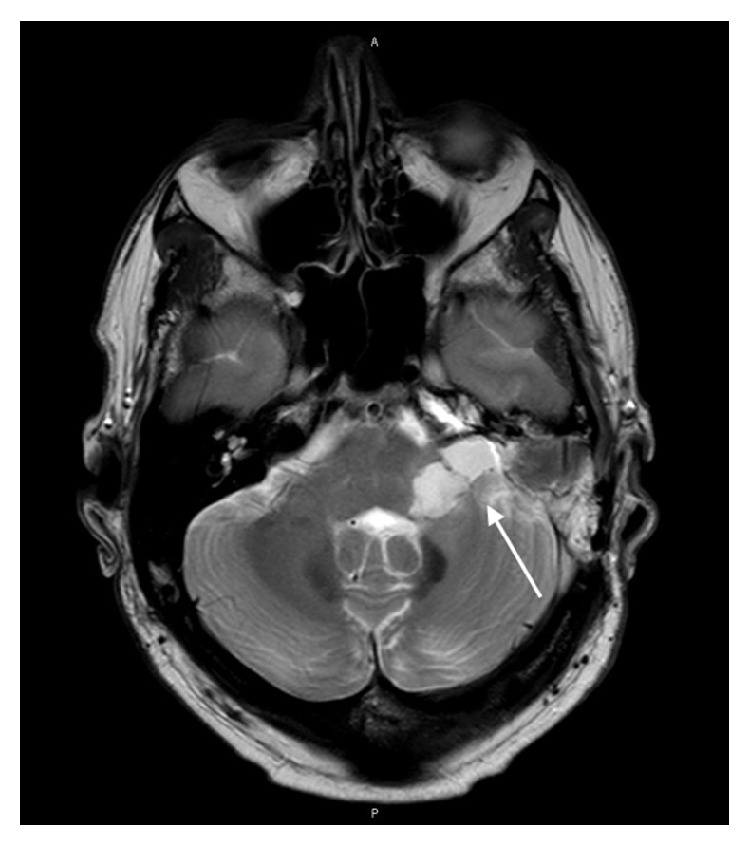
Axial MR image (T2 weighted) showing the postoperative changes.
